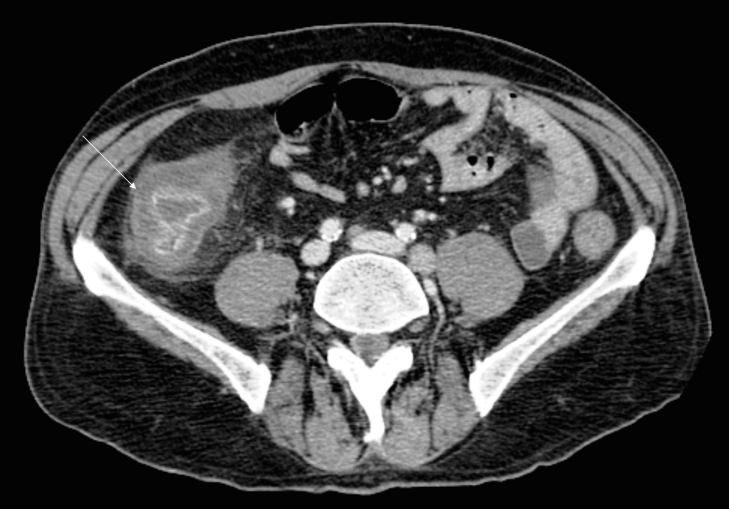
Post-contrast axial CT image, showing hypodense mural thickening of the caecum (arrow), with irregular contrast enhancement of the endoluminal surface.
